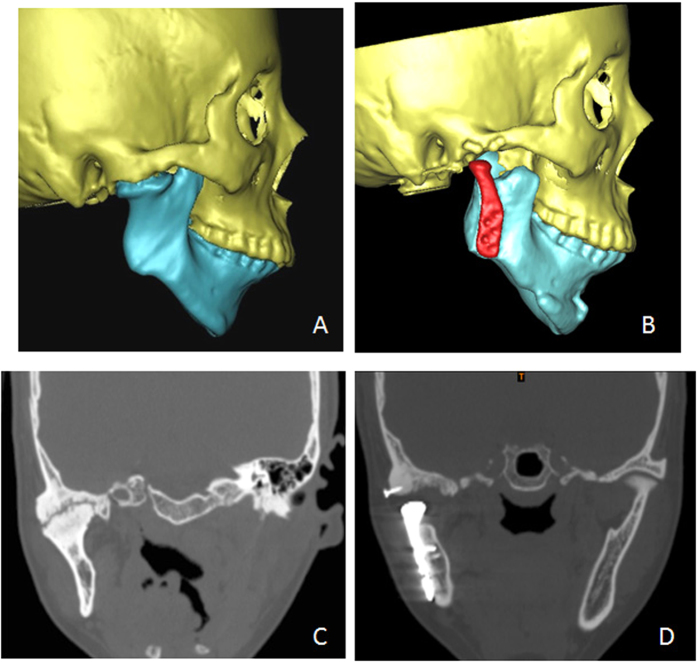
CT images of Patient 7. (A) Pre-operative 3D CT; (B) Post-operative 3D CT; (C) Pre-operative CT coronal reconstruction; (D) After 23 months, no relapse of ankylosis can be seen in CT coronal reconstruction.
